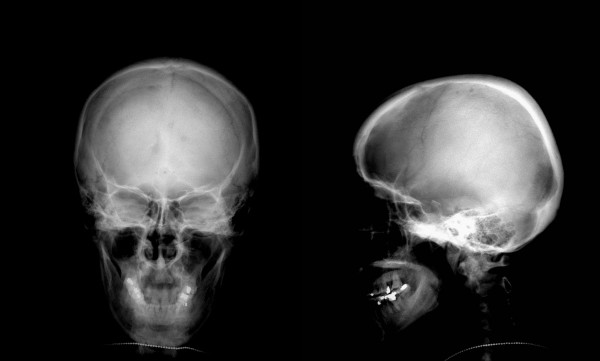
X-ray revealed low density foci on the skull(posterior-anterior and lateral film).
